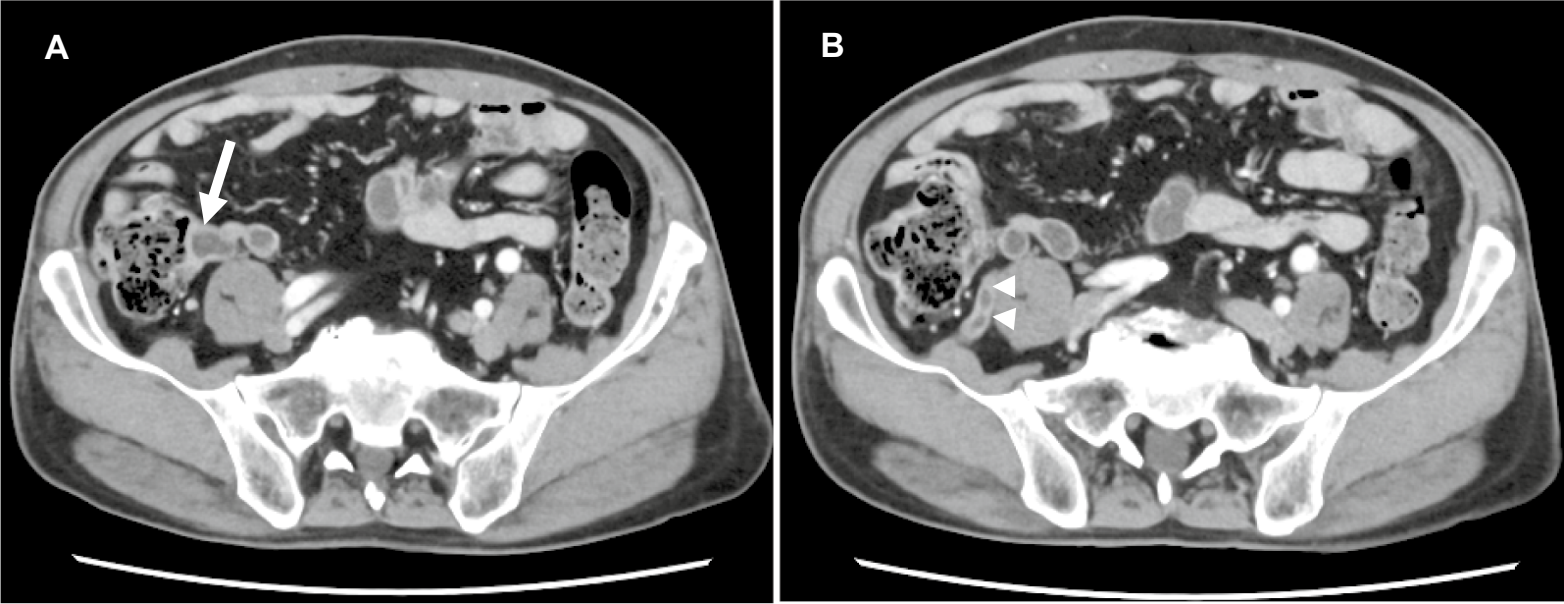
Abdominal computed tomography (CT) scan. Enhanced abdominal CT demonstrated a cecal cystic mass (a, arrow) without significant lymph node enlargement in the abdominal cavity. The appendix was almost normal size and do not have wall thickness or an appendicolith, but had a slightly dilation of the lumen (b, arrowheads)
